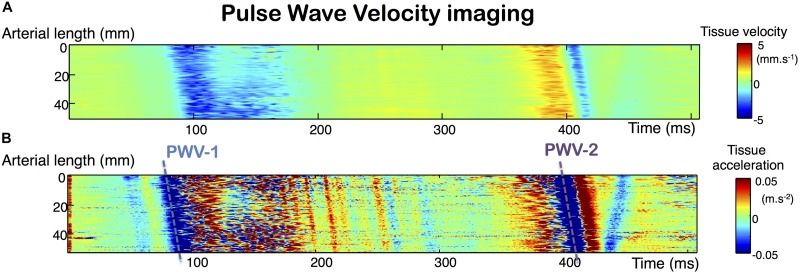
Ultrafast tissue Doppler imaging along the arterial wall over the cardiac cycle. Analysis of the tissue Doppler velocities of the arterial walls over time provides access to pulse wave velocity (PWV) measurements (A). The measurement is obtained by the automated calculation of the slope of the main tissue Doppler acceleration peaks (B).
